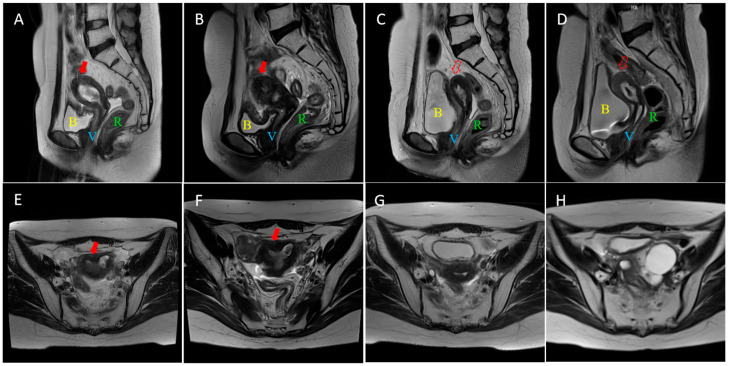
Pelvic MR images after recurrence. Representative sagittal and cross-sectional MR images of uteri fundus at recurrence (July 2022, (A,E)), after salvage EBRT/before ISBT (September 2022, (B,F)), 3 months after salvage RT (February 2023, (C,G)) and 37 months after salvage RT (May 2025, (D,H)). The red arrow indicates the recurrent cancer in the uterus fundus, and the red dash-line arrow indicates the recovered uterus fundus. B in yellow color: Bladder, V: Vagina, R: Rectum.
